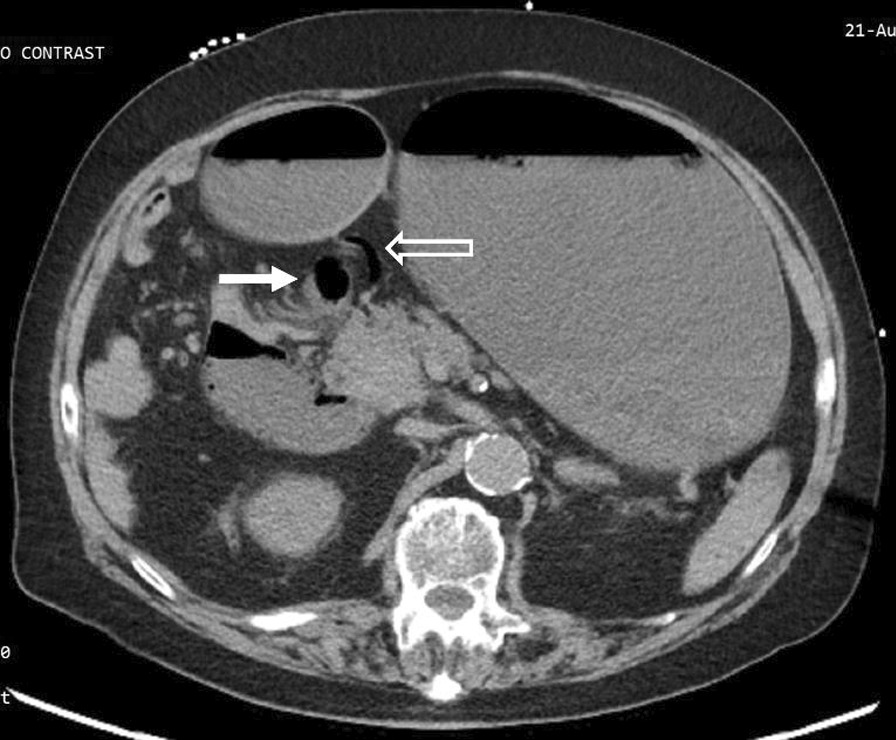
Right paraduodenal hernia. CT of the abdomen without contrast. Transition point (solid arrow) at the third segment of the duodenum, posterior to the ascending mesocolon in the expected location of the fossa of Landzert. Suggestive of a right paraduodenal hernia. Incidentally noted gas in a superior mesenteric vein tributary (hollow arrow)
